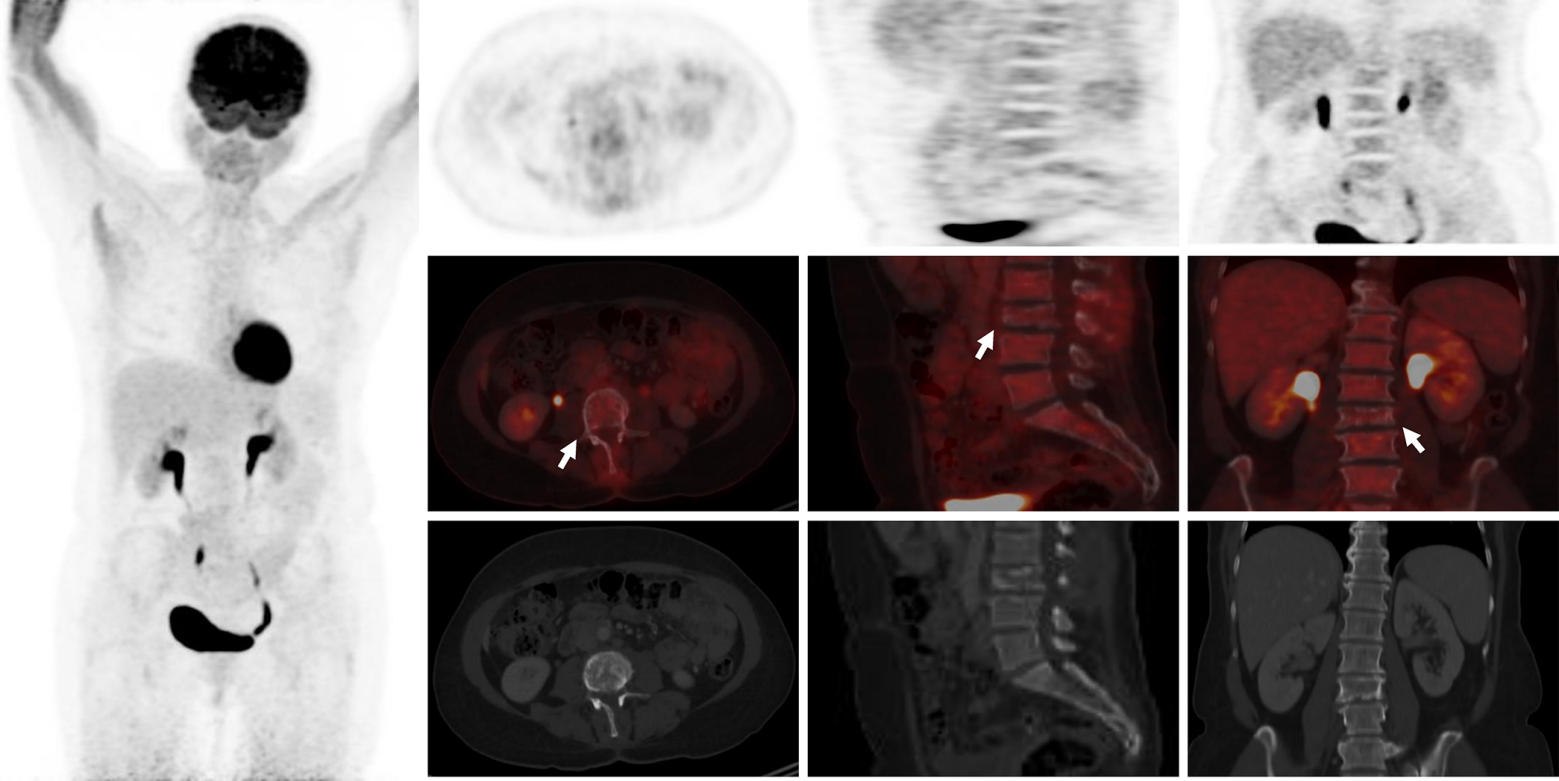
^18^F-FDG-PET/CT showing complete metabolic response/normalization at L3 vertebra level and the L3–L4 intervertebral disc after 4 cycles of chemotherapy (white arrows).
